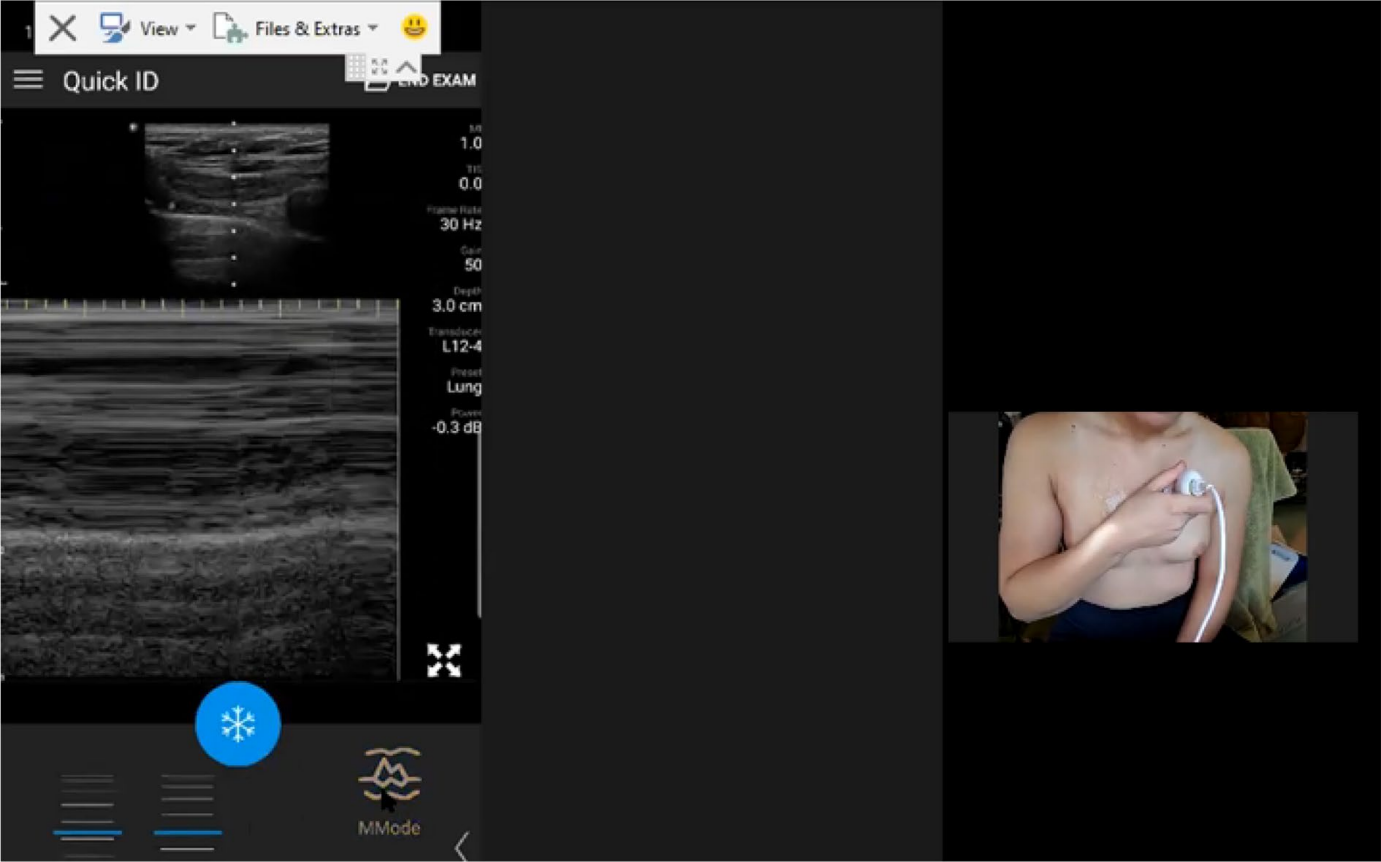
First follow-up Self-Performed Lung Ultrasound Study of 31-year-old potentially exposed patient-spouse 2 weeks after patients COVID-19 Diagnosis. Screenshot of the Remote Mentors Computer Screen that shows the patient imaging herself to the right of the screen and the resultant ultrasound image showing the “seashore” sign to the left. As lung ultrasound is a dynamic real-time examination hard to capture in any single image, readers are strongly encouraged to view the accompanying supplemental videos of the entire examination online to gain a true appreciation of the technique
